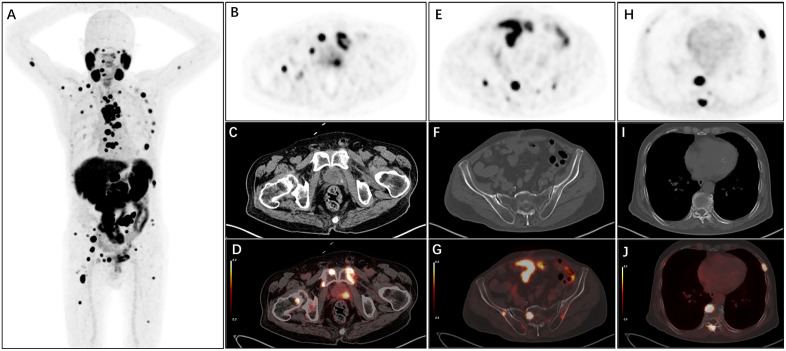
[¹⁸F]PSMA-1007 PET/CT imaging of prostate bed recurrence with multi-site osseous metastatic castration-resistant prostate cancer. This imaging was acquired in a 71-year-old male with castration-resistant prostate cancer at 40 months post-radical prostatectomy. The patient presented with a PSA level of 8.7 ng/mL, and the primary tumor was pathologically graded as Gleason score 8 (ISUP Grade 4). (A) Whole-body MIP of [¹⁸F]PSMA-1007 PET demonstrates diffuse [¹⁸F]PSMA-1007-avid lesions involving the pelvic region and multiple skeletal sites throughout the body. Axial PET (B, E, H), CT (C, F, I), and fused PET/CT (D, G, J) images confirm the following multi-focal lesions: Recurrence and metastatic lesions in the prostate bed, bilateral pubic bones, right ischium, and femoral neck (B–D); Osseous metastases in the sacrum and bilateral iliac bones (E–G); Osseous metastases in the thoracic vertebrae and bilateral ribs (H–J).
